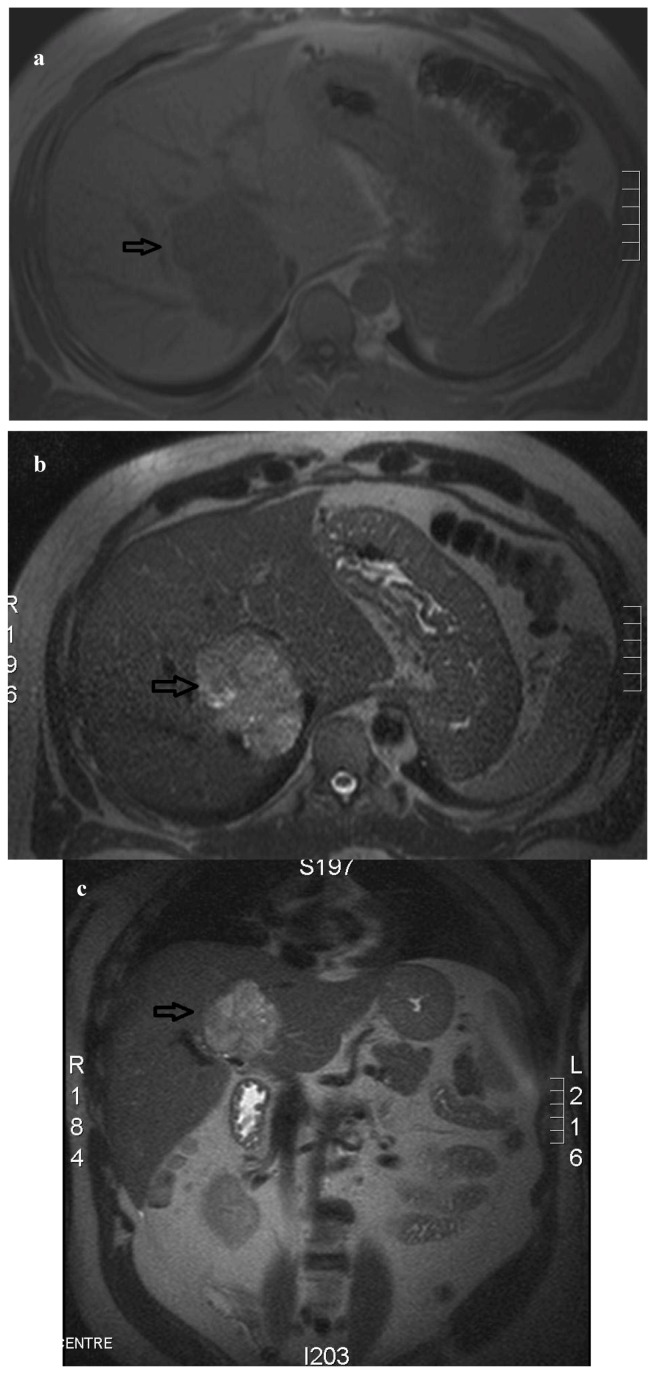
(a) An axial T1-weighted MRI of the abdomen demonstrates a solitary lesion (arrow) in the right lobe of the liver, axial. (b) T2-weighted MRI shows a single hyperintense liver lesion (arrow). (c) T2-weighted MRI coronal views.
